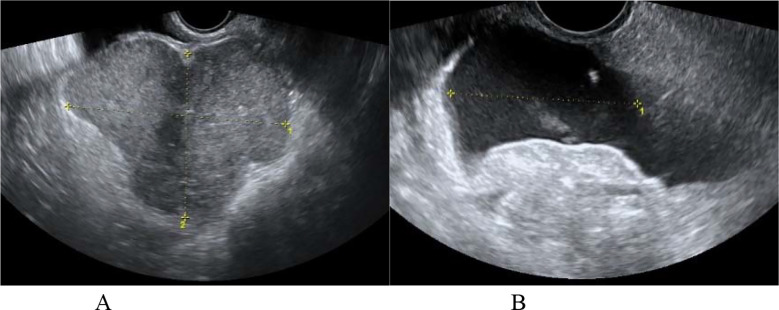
A patient with right type III PFTC (No. 15). (A): the transvaginal two-dimensional ultrasound showed the lobulated solid mass in left side of pelvic cavity, with no abnormal mass found in the right adnexal region; (B): the ultrasound showed the pelvic fluid accumulation.
